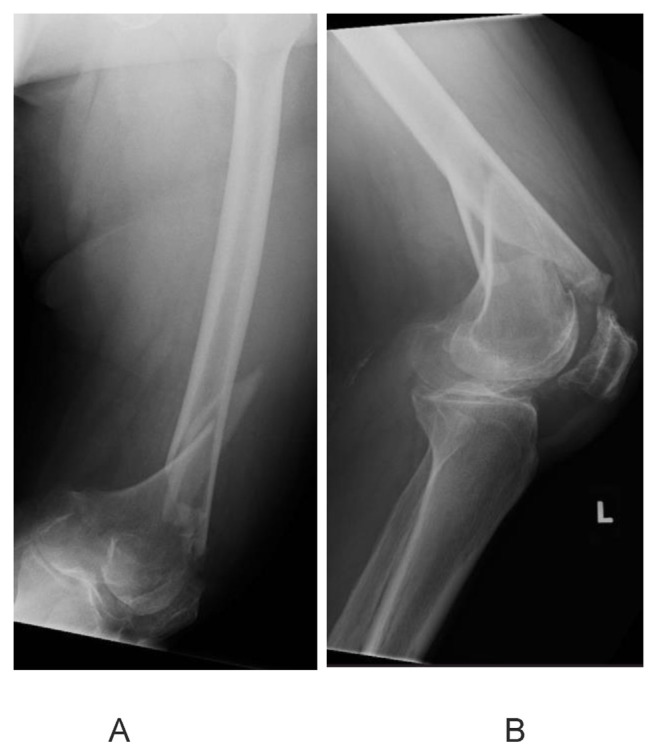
Preoperative radiographs show a comminuted, extra-articular fracture of the left distal femur.
